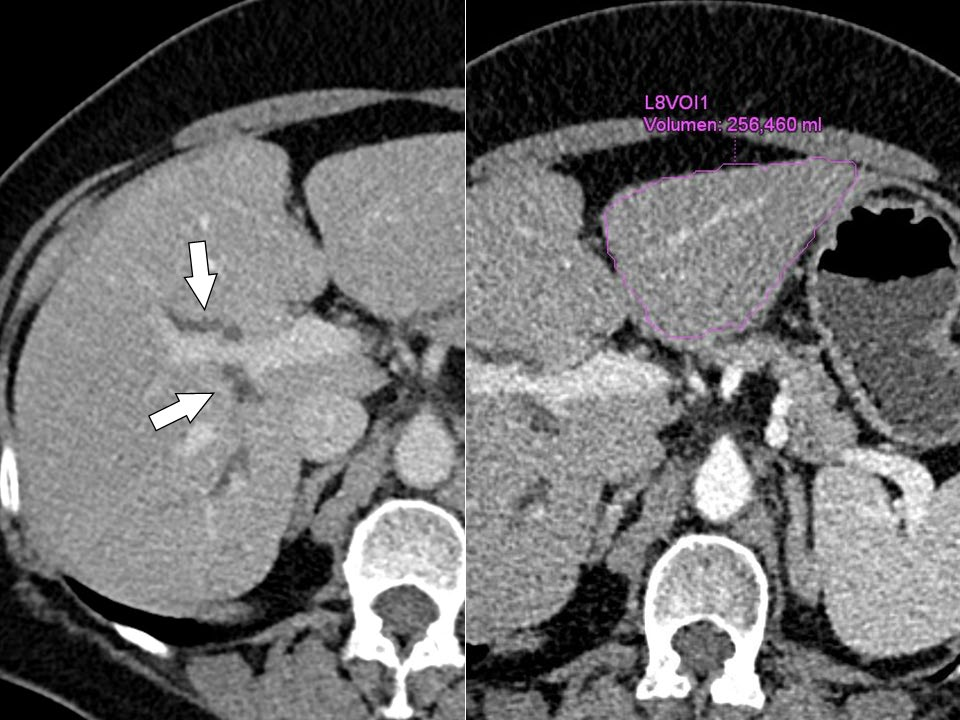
Contrast-enhanced MDCT (left) shows segmental biliary duct dilatation (arrows) in the right lobe, without demonstration of a tumor; (Right) of note is the small liver segments 2/3 with a volume of 256 mL. MDCT: multidetector CT
